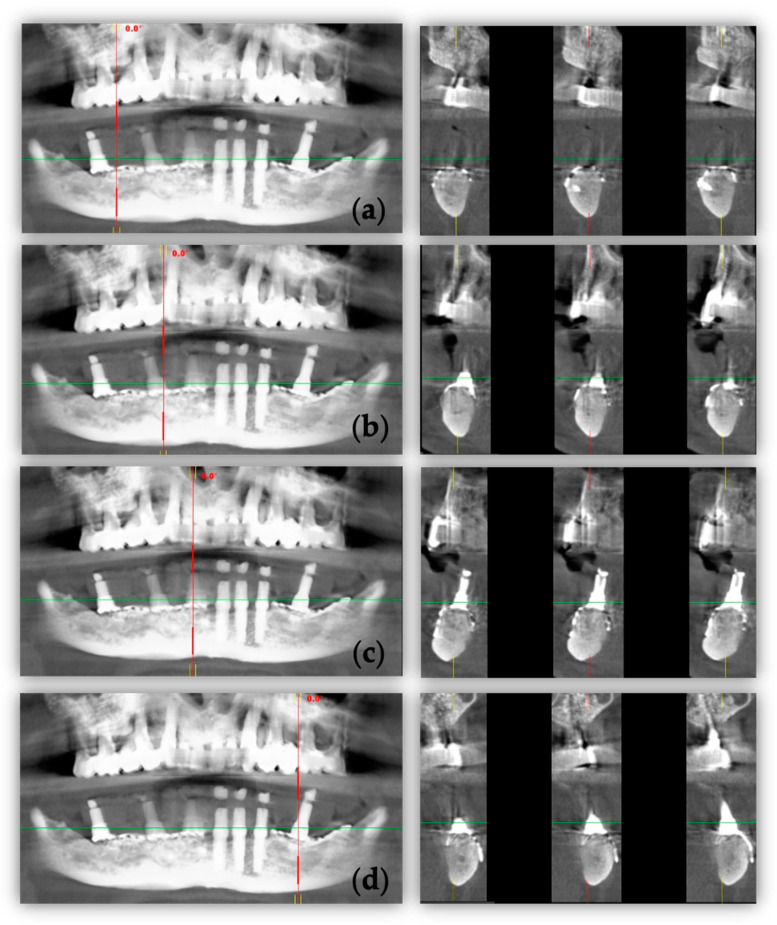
Six-month postsurgical CBCT images: (a–d) panoramic (green lines) and cross-sectional (red lines) views.
